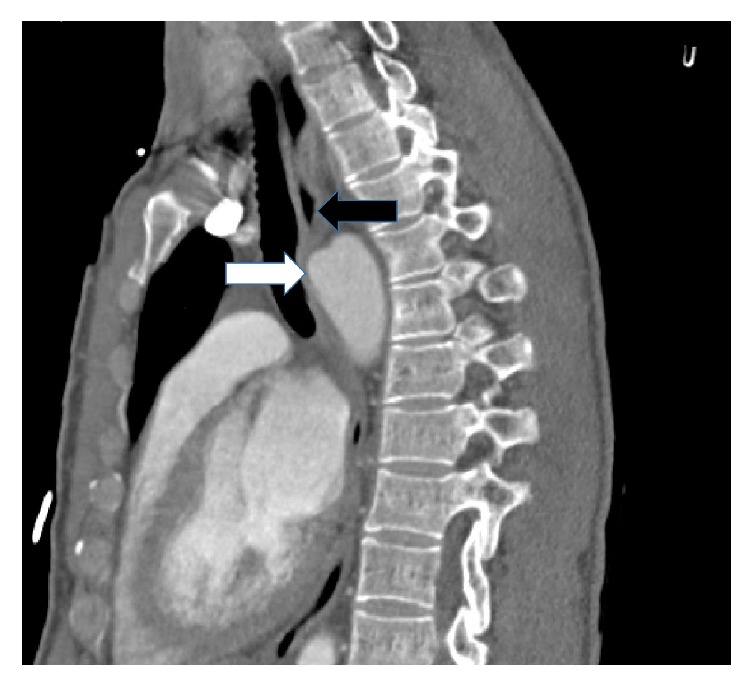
High resolution CT angiogram, sagittal view showing the ductus diverticulum (white arrow) indenting the esophagus (black arrow).
